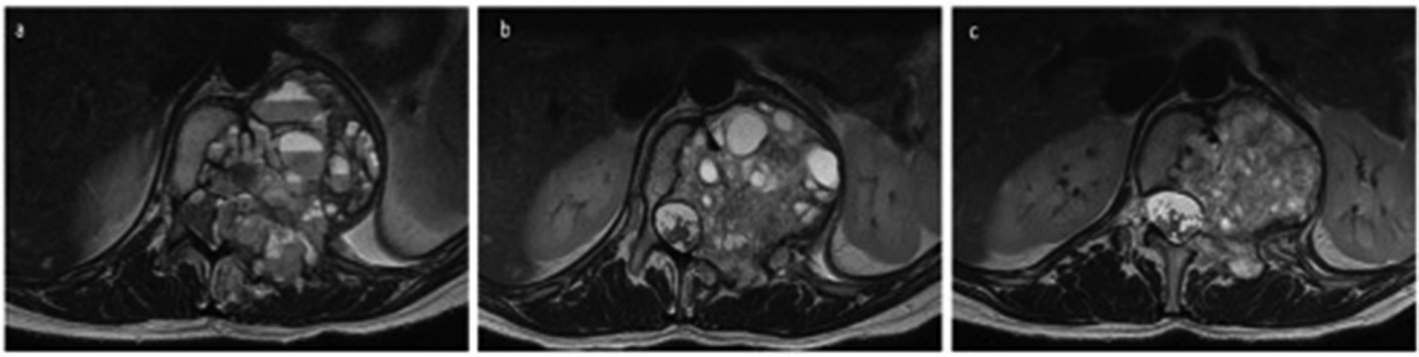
(a–c) MRI of patient pre-treatment, 4 months post-treatment and 9 months post-treatment respectively in sequential order. (b, c:) Axial T2 sequence at T12 level demonstrates interval decrease in size of lesion with little or no epidural involvement. There is now normal CSF spaces with no compression against the cord. CSF, cerebrospinal fluid fluid.
